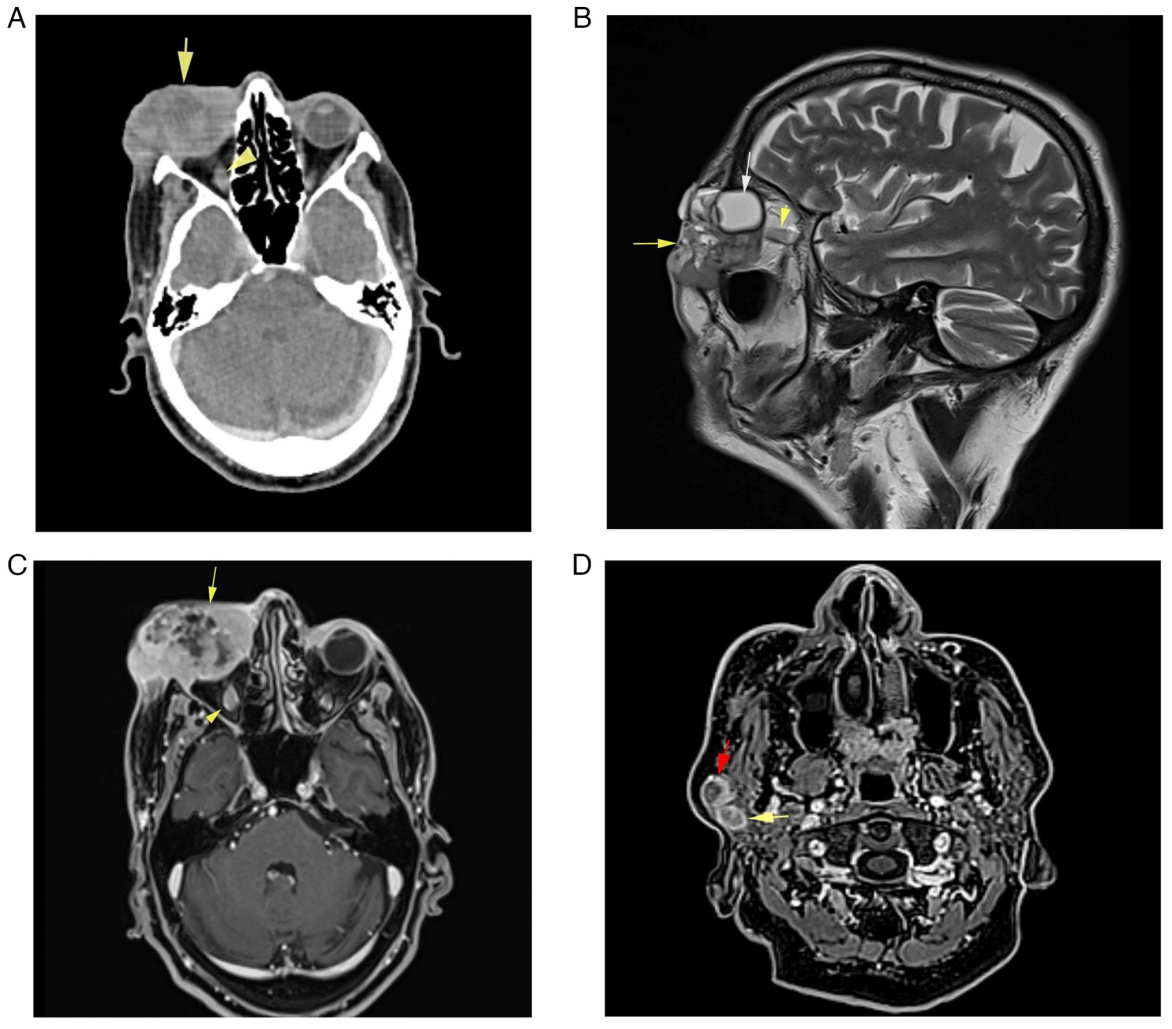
Pre-treatment imaging revealing a significant right periorbital mass. (A) An axial contrast-enhanced computed tomography scan of the face and skull exhibited a large, well-defined mass (arrow) and the optic nerve (arrowhead). (B) A sagittal T2-weighted image revealed a large cystic-solid lesion (yellow arrow) with orbital (white arrow), periorbital and intraorbital extension. The optic nerve appeared free of any invasion (yellow arrowhead). (C) Post-contrast axial T1 fast suppression imaging showed a large, enhanced lesion (arrow) with foci of cystic degeneration represented by non-enhancing areas and the optic nerve (arrowhead). (D) Pre-treatment axial fat-suppressed T1-weighted images revealed two oval-shaped lymph nodes within the parotid gland, exhibiting peripheral enhancement and central necrosis (yellow and red arrows).
